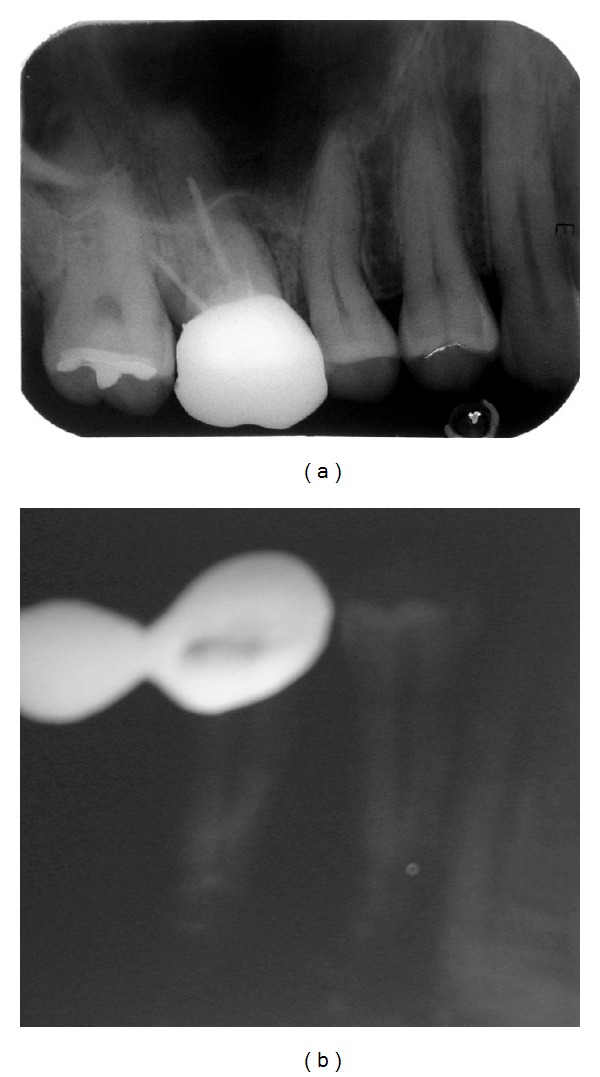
(a) Photograph of intraoral periapical radiograph of 14 showing normal root morphology. (b) Photograph of intraoral periapical radiograph of 44 showing normal root morphology.
